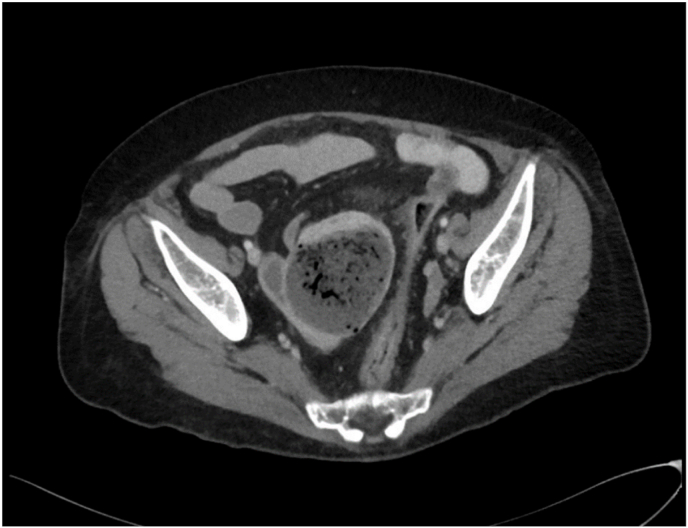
Pre-operative CT findings.
